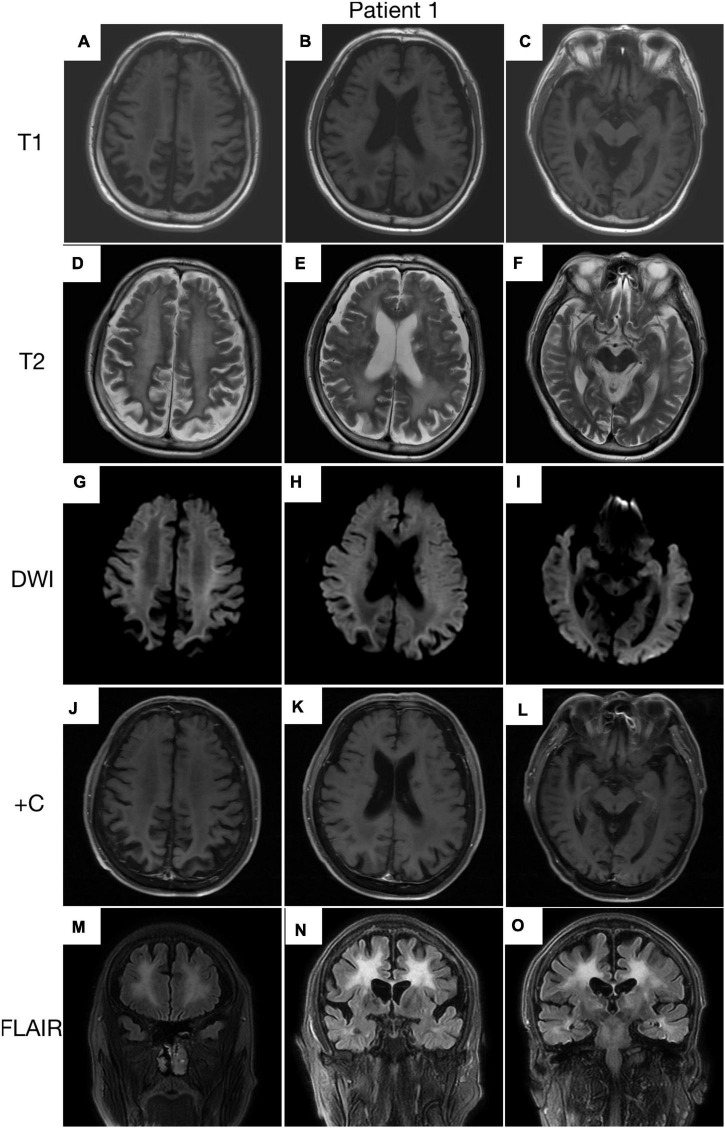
Brain MRI scan of patient 1. Cerebral atrophy was observed on T1 images (A–C). T2 (D–F) and FLAIR (M–O) images showed severe leukoencephalopathy. Diffusion-weighted imaging (DWI) images revealed high linear signal in the corticomedullary junction (G–I). No significant enhancing lesions were observed (J–L).
